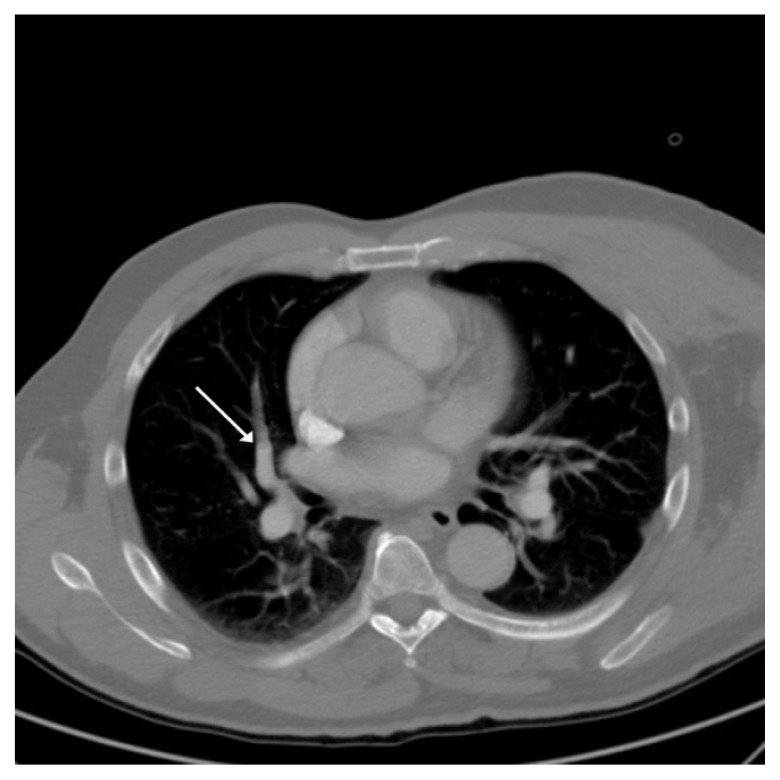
CT angiogram showed a PE involving the medial segment of the right middle lobe of lung.
